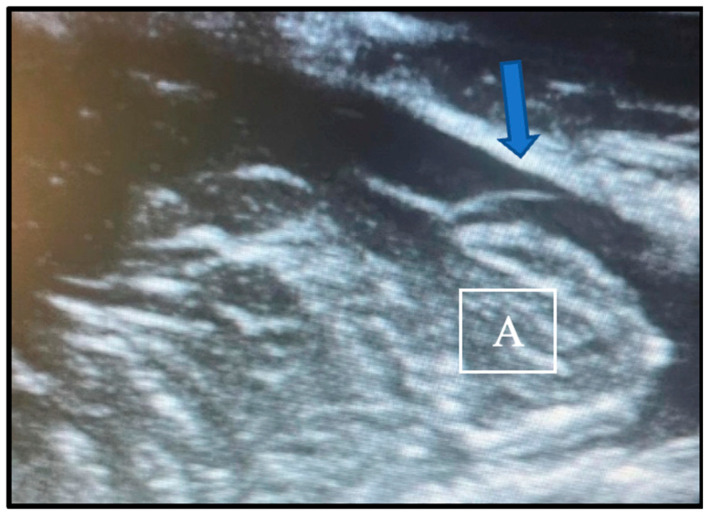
View of intraoperative ultrasound images depicting the absence of CSF flow (arrow) behind the cerebellar tonsils (A), indicative of the inadequate decompression of the structures.
